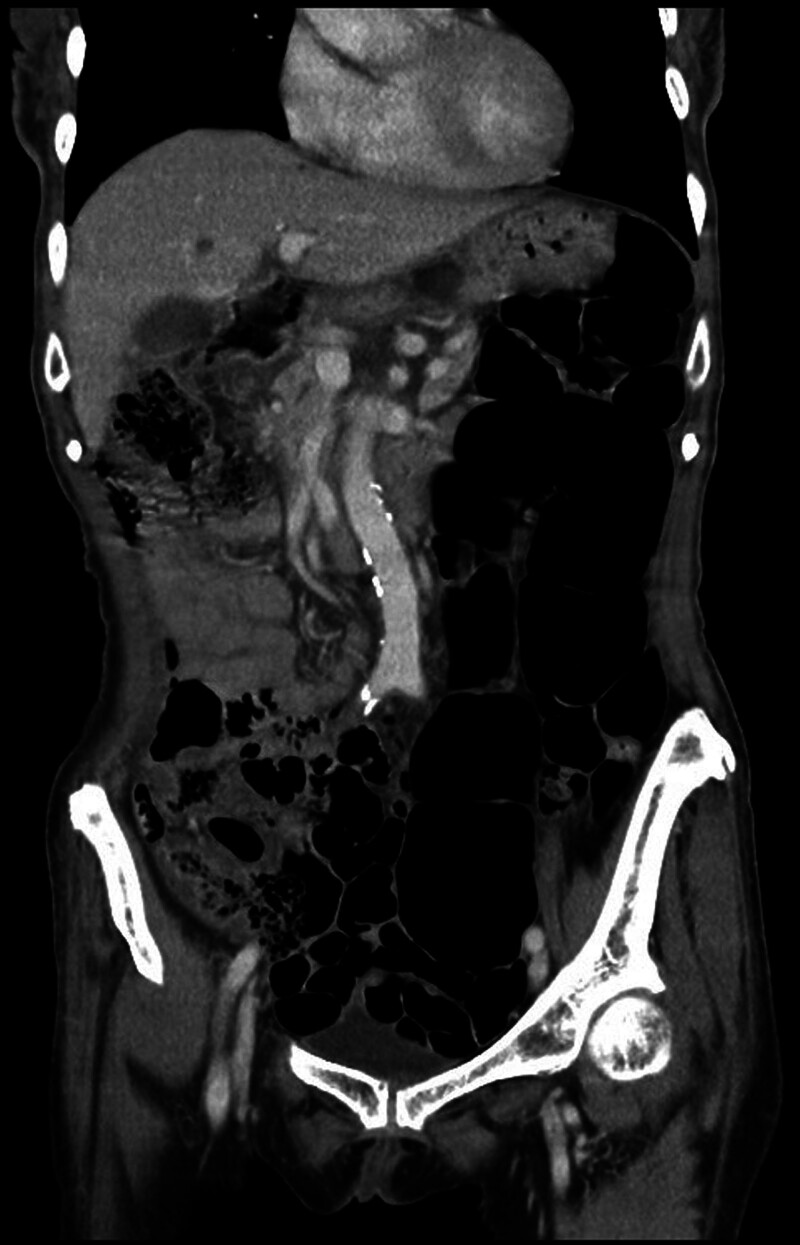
An abdominal computed tomography of this patient.
